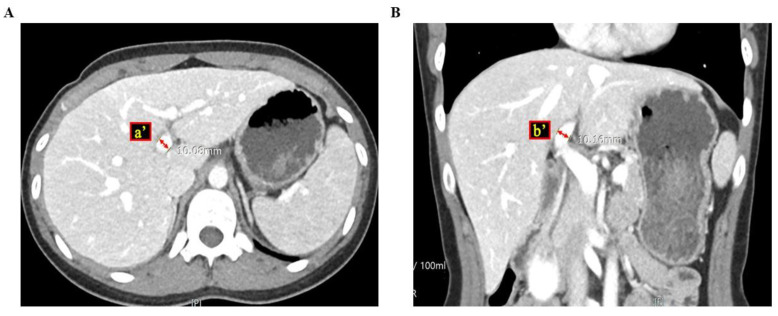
These figures show how to measure the cross-sectional area of the left portal vein via computed tomography ((A) cross section view; (B) coronary section view), where a’ and b’ are the semi-major and minor axes of the left portal vein.
